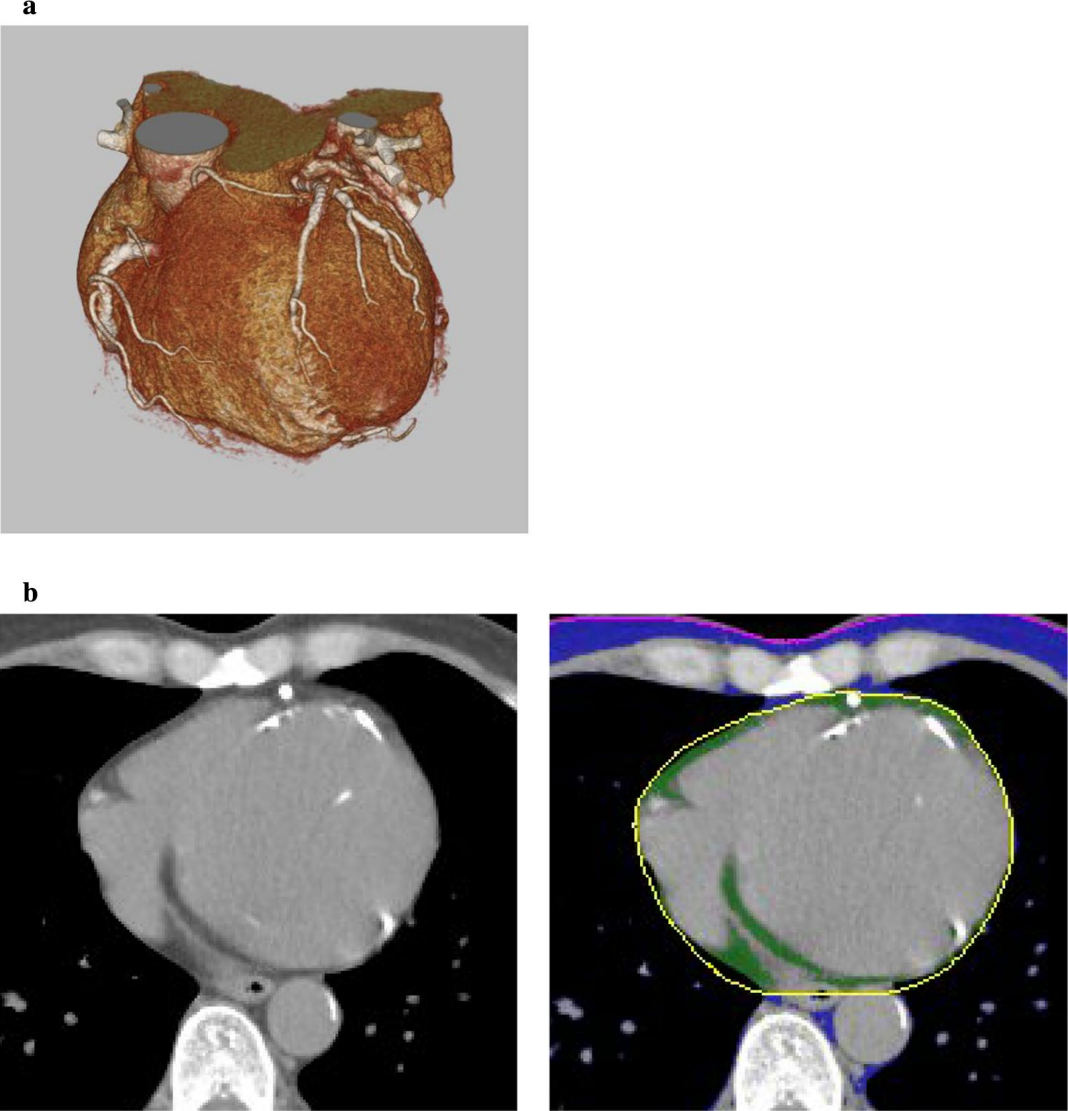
Measurement of epicardial adipose tissue (EAT) volumes with multislice CT. a 3D image. EAT area was measured on each axial image from the lower surface of left pulmonary artery origin to the left ventricular apex, and total EAT volume was obtained from multiplying total EAT areas by the slice thickness. b Axial images. A region of interest (ROI) was manually placed along the visceral pericardium (left and middle panel). EAT was extracted on an axial image (green) (right panel)
